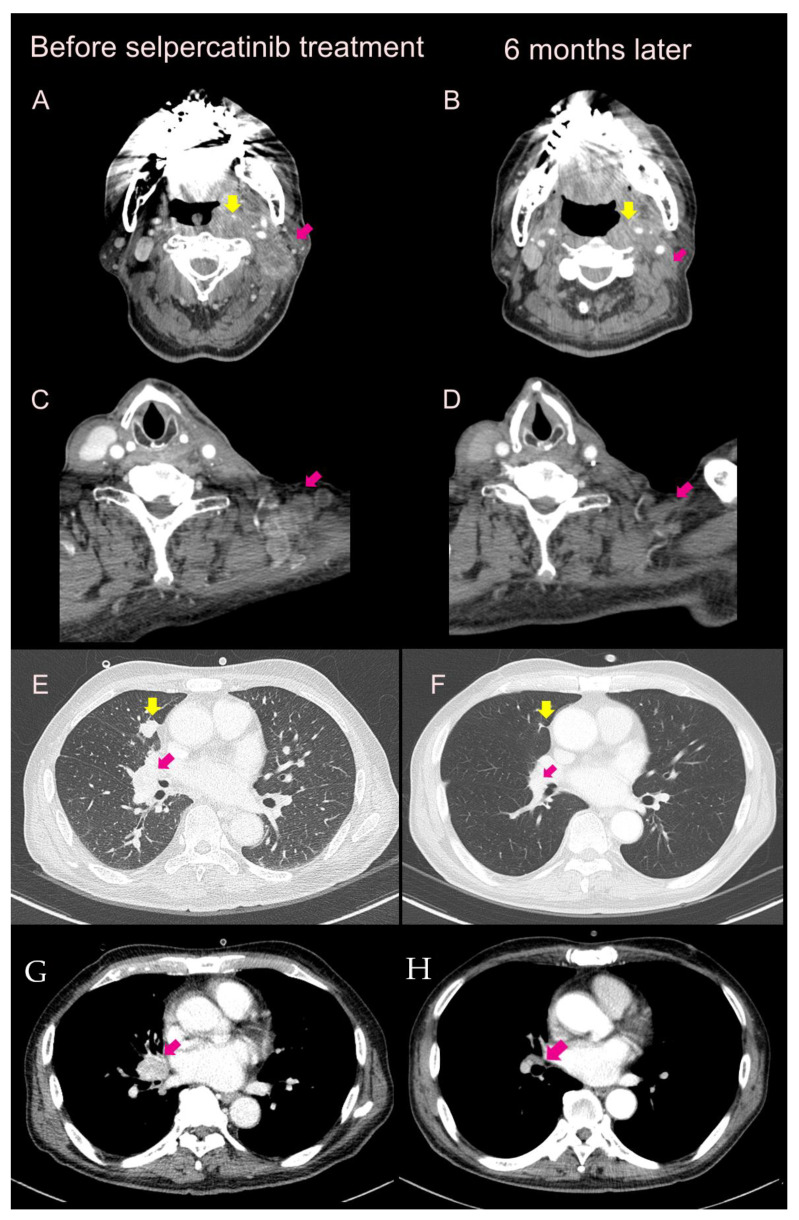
CT scan images of the Case 1 patient before and at six months after selpercatinib treatment. Before selpercatinib treatment, there were metastatic lesions at the neck (A); lateral lymph node (C); lung parenchym (E); and hilar lesion (G). All of them showed regression at six months after selpercatinib treatment (B,D,F,H) (yellow and purple arrows).
